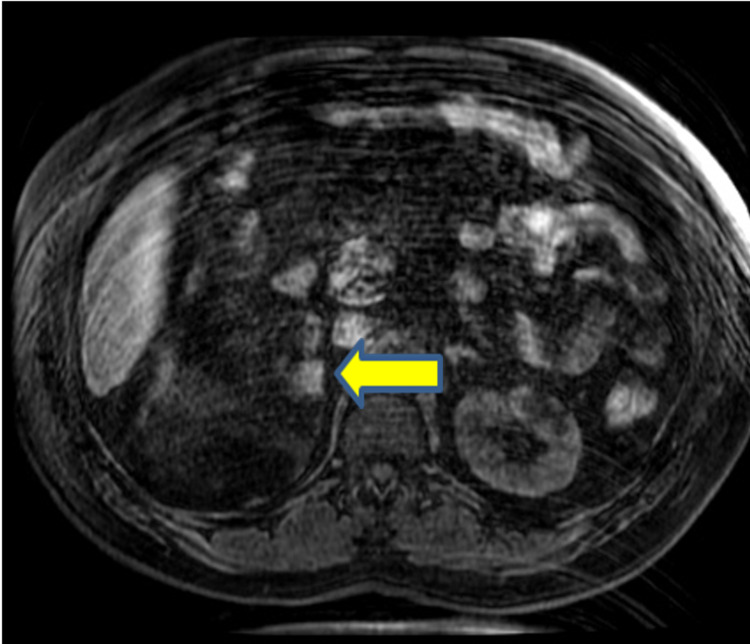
MRI Pre T1 Fat Saturated - Axial View Image shows foci of high signal indicating hemorrhage (yellow arrow).
